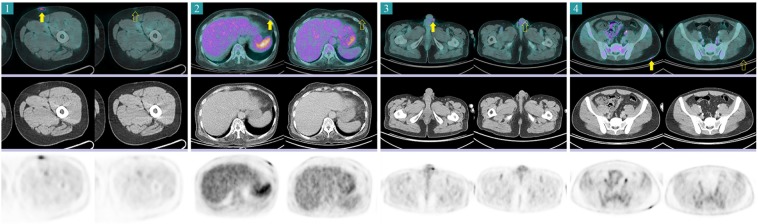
Eschar lesions from 4 patients. Before antibiotic treatment (each left columns), eschar lesions appeared as hypermetabolic skin thickenings. Skin thickness and FDG uptake were markedly decreased after treatment (each right columns). The yellow arrows indicate the pretreatment eschar lesions, and the yellow empty arrows indicate the same sites after treatments.
